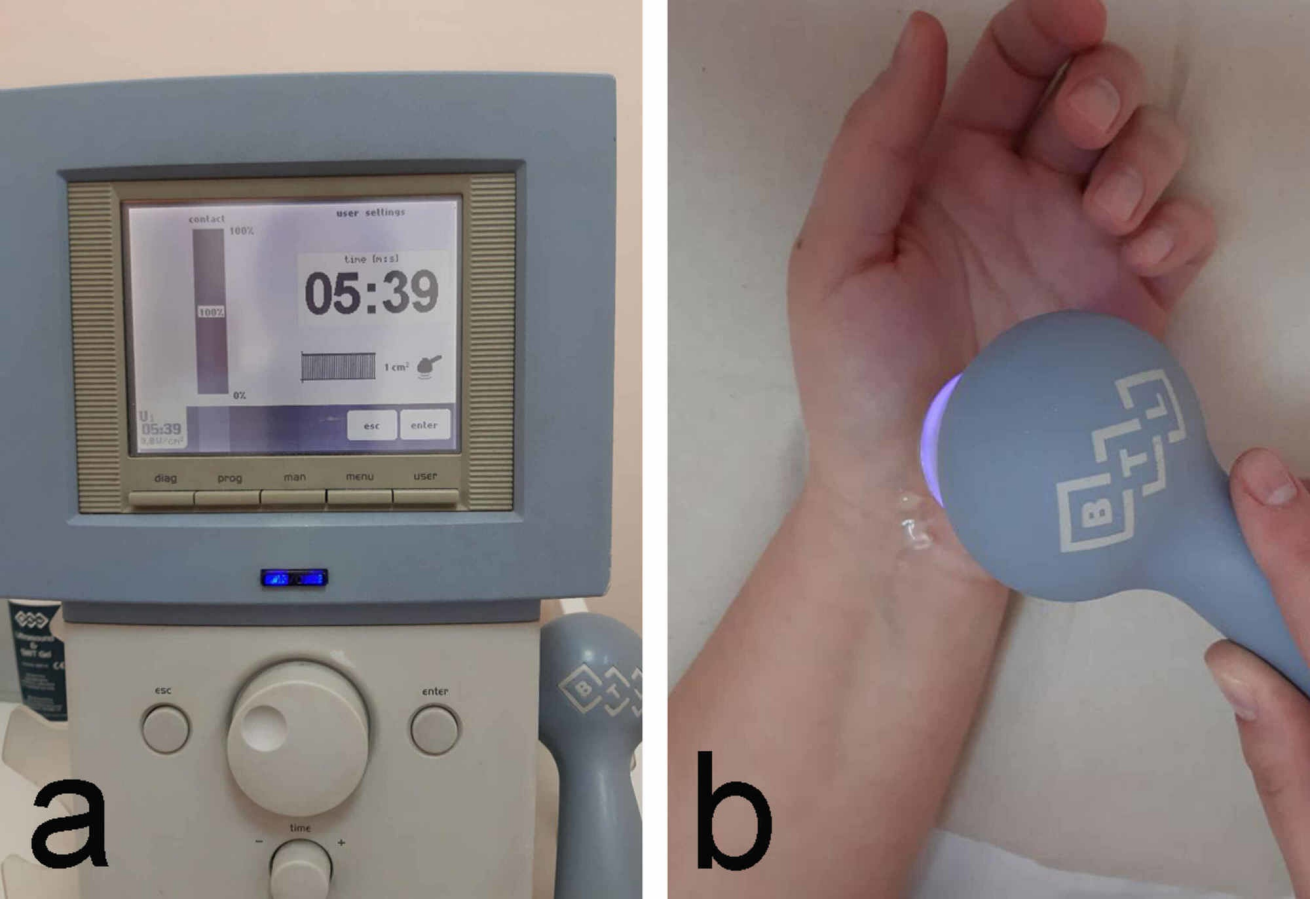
Therapeutic ultrasound a: device; b: application technique
